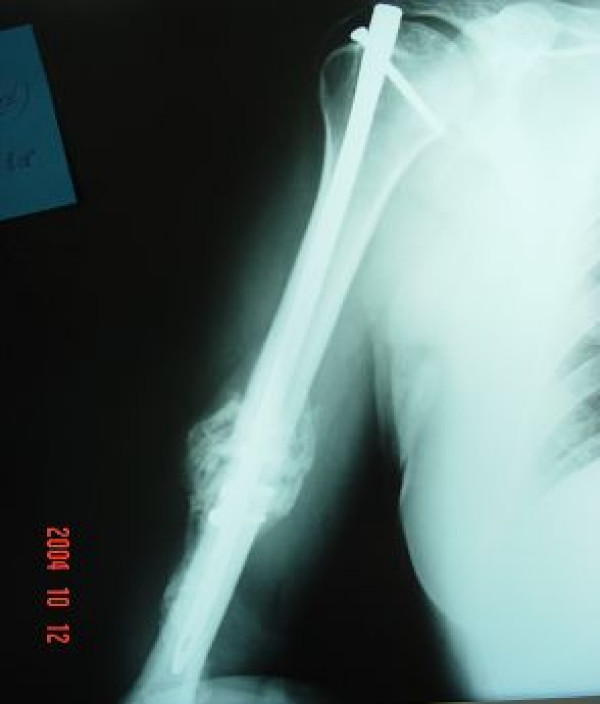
Radiograph showing union of the fracture at 12 weeks postoperatively.
